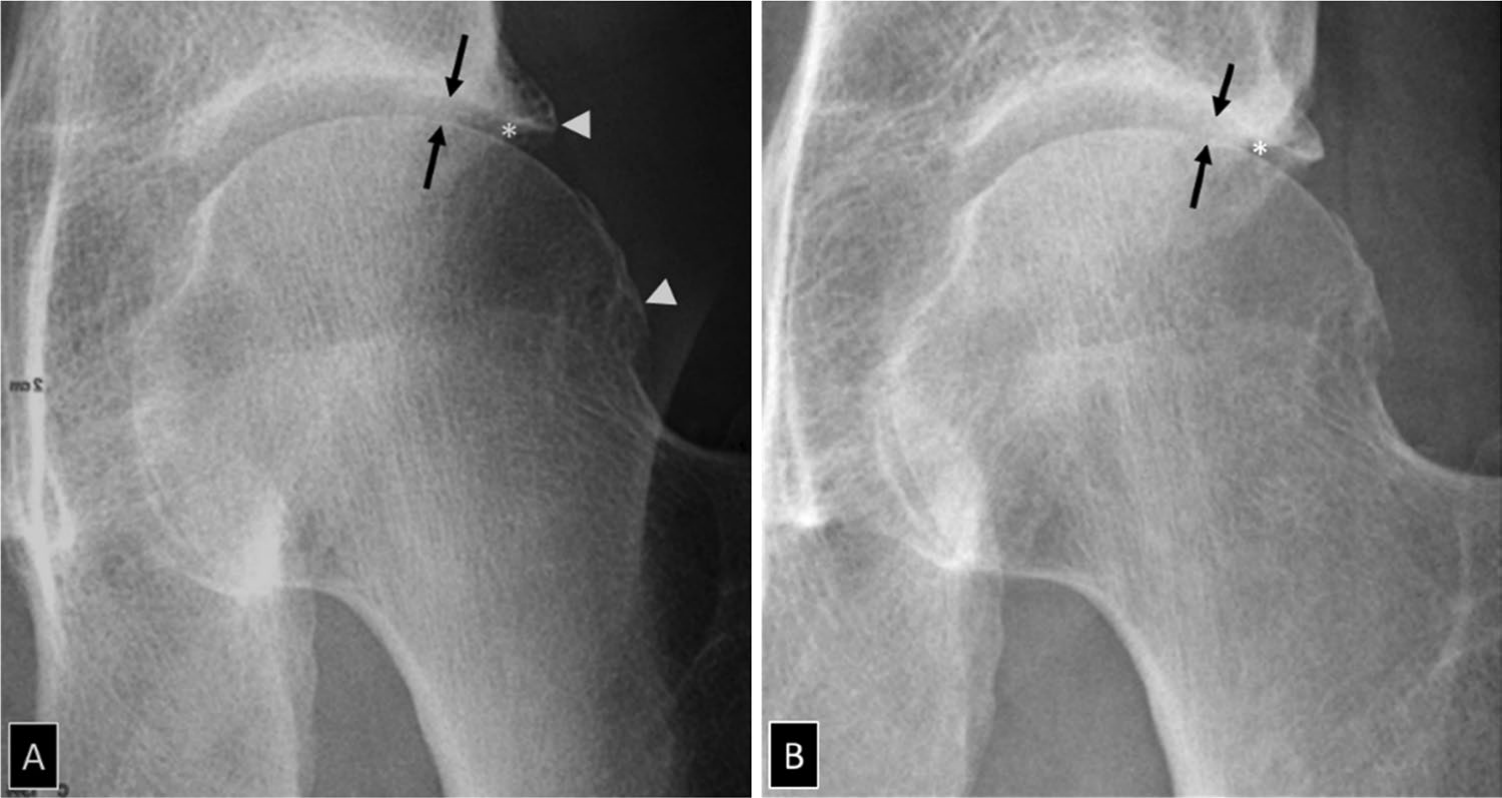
Non-evolutive osteoarthritis of the left hip in a 62-year-old man with limited range of motion but no hip pain. A AP radiograph of the left hip demonstrates lateral joint space narrowing (arrows) and marginal osteophytes (arrowheads). B Follow-up radiograph after 10 years demonstrates no significant change in joint space narrowing. Outcome prediction on radiographs is unreliable. Note that the area underneath the osteophyte (asterisk) does not correspond to the articular joint space
